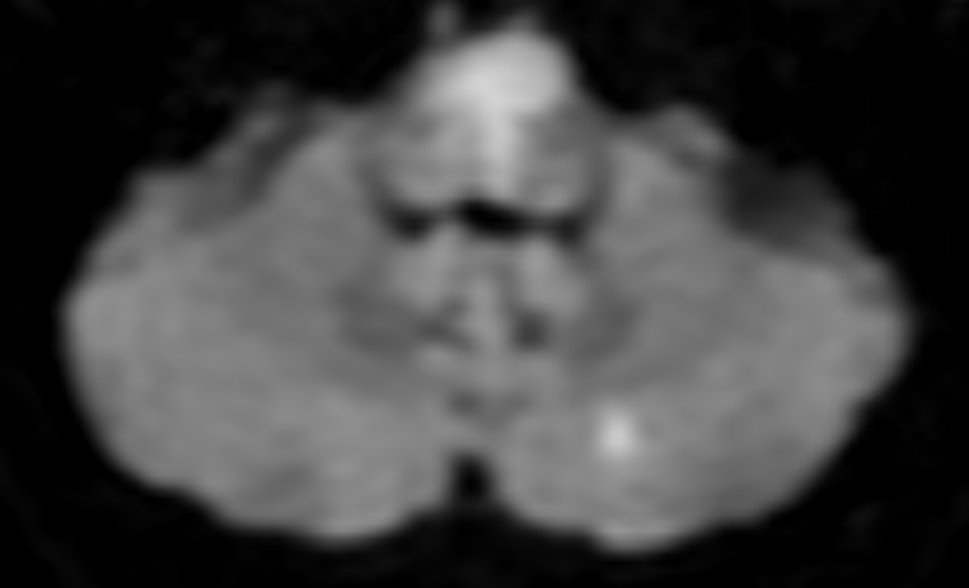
DWI MRI showing the typical heart-shaped appearance of bilateral medial medullary infarcts. There is also a small infarct in the left posterior inferior cerebellar artery territory
